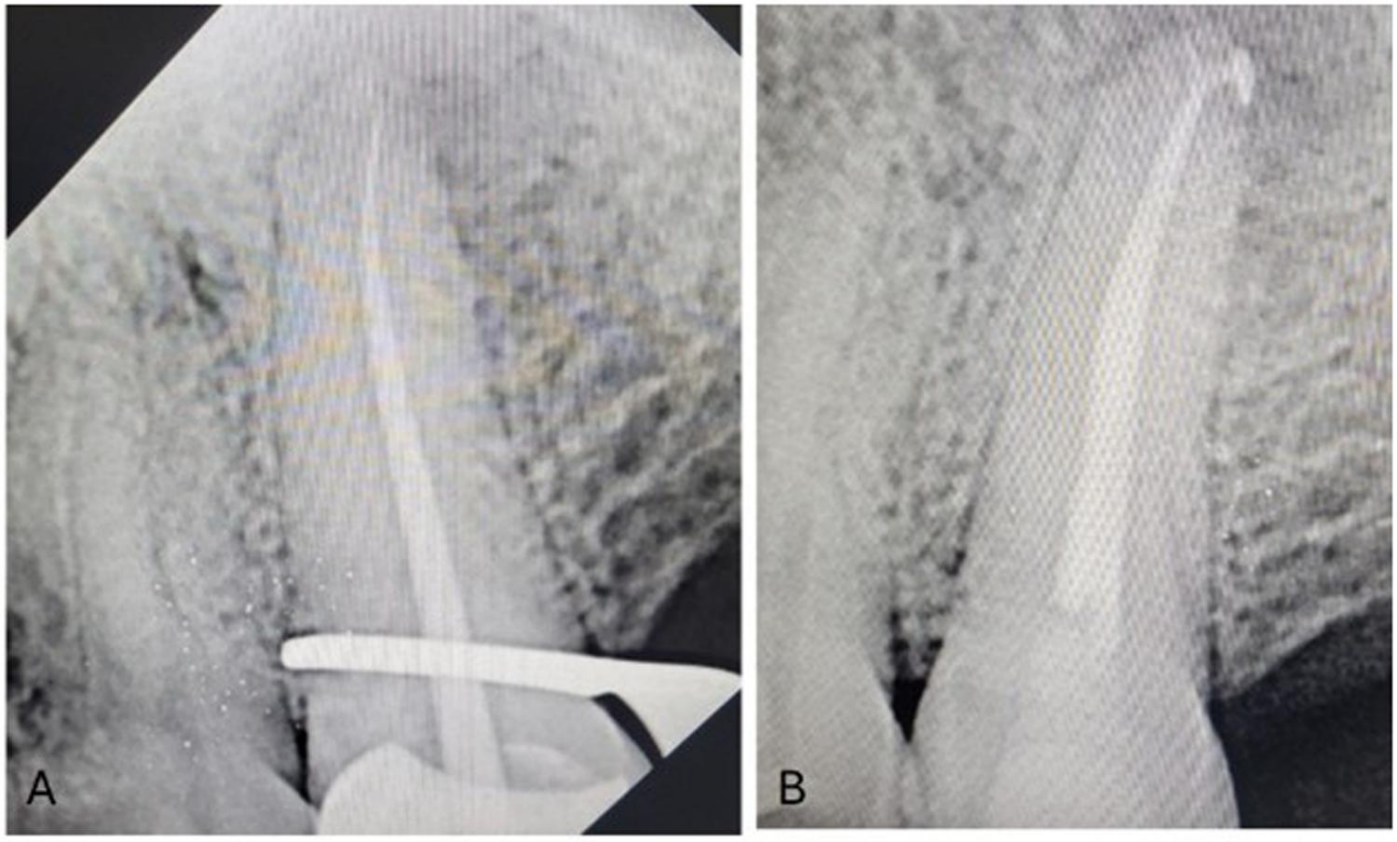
(A) Preoperative IOPA radiographic representation of pulp space therapy with the use of bioceramic sealer. (B) Postoperative IOPA radiographic representation of pulp space therapy with the use of bioceramic sealer.
